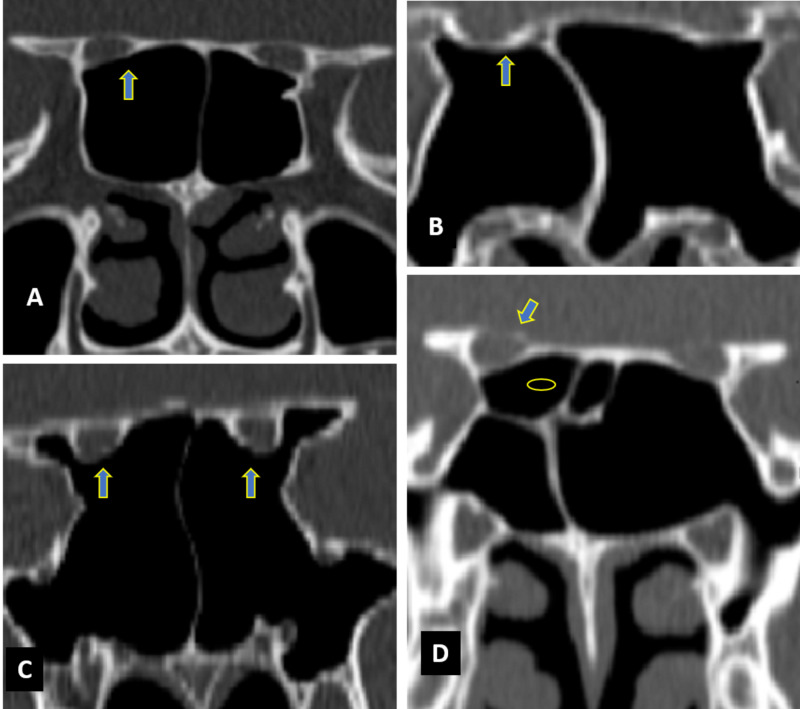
Coronal CT images of paranasal sinuses showing optic nerve variations (arrows) Optic nerve (A) type I bilaterally, (B) type II bilaterally, (C) type III bilaterally, (D) type IV on the right side and type I on the left side (oval indicates posterior ethamoidal sinus).
